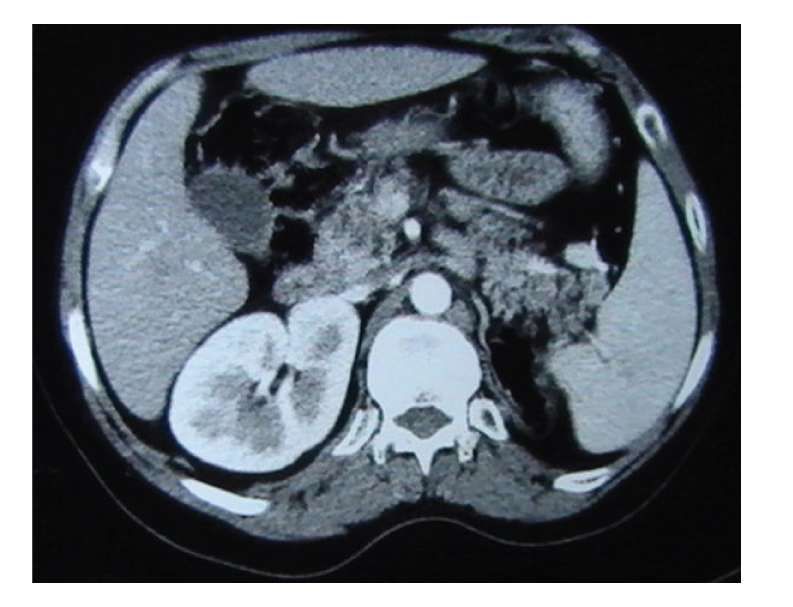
Abdominal CT: unilateral left renal aplasia.
